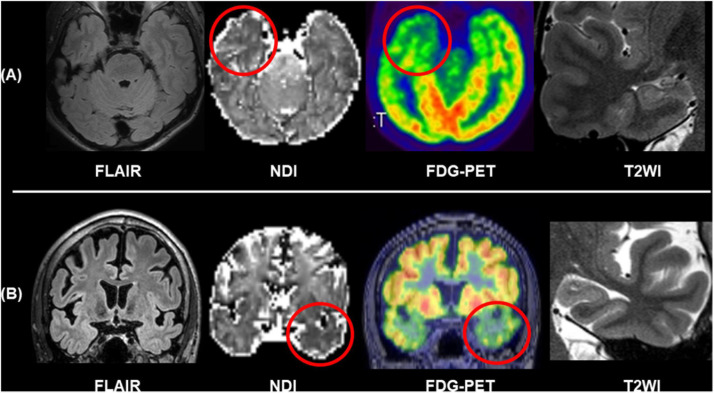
Two cases of conventionally MRI-negative PET-positive TLE. While no abnormalities were found in FLAIR and T2WI including the hippocampus, neurite orientation dispersion and density imaging (NODDI) revealed reduced neurite density of the focus side. (A) Modified from Sone et al. (2018). (B) Modified from Sone (2019).
